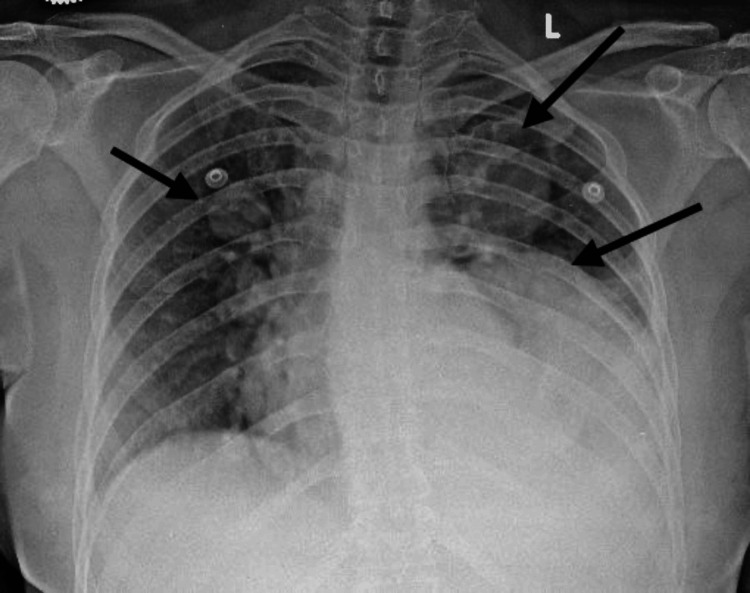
Chest radiograph showing bilateral well-defined, homogeneous opacities (arrows)
